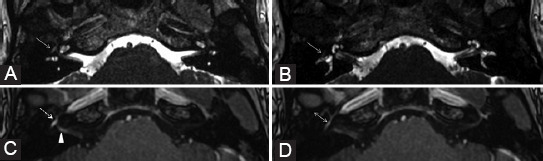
A 28-year-old patient with previous surgery for the right parotid pleomorphic adenoma (with facial nerve preservation) presents for the right peripheral facial nerve paralysis with rapid onset, biologically with inflammatory syndrome. Axial 3D FIESTA images (A, B) show a slight signal hyperintensity at the level of the tympanic segment (arrow) of the right CN VII. The contrast-enhanced 3D T1-weighted images (C, D) demonstrate moderate uniformly linear CE of the right geniculate ganglion (dashed arrow), canal (arrowhead), and tympanic (double arrow) segments, more intense than the normal left side. The symptoms improved after the treatment.
